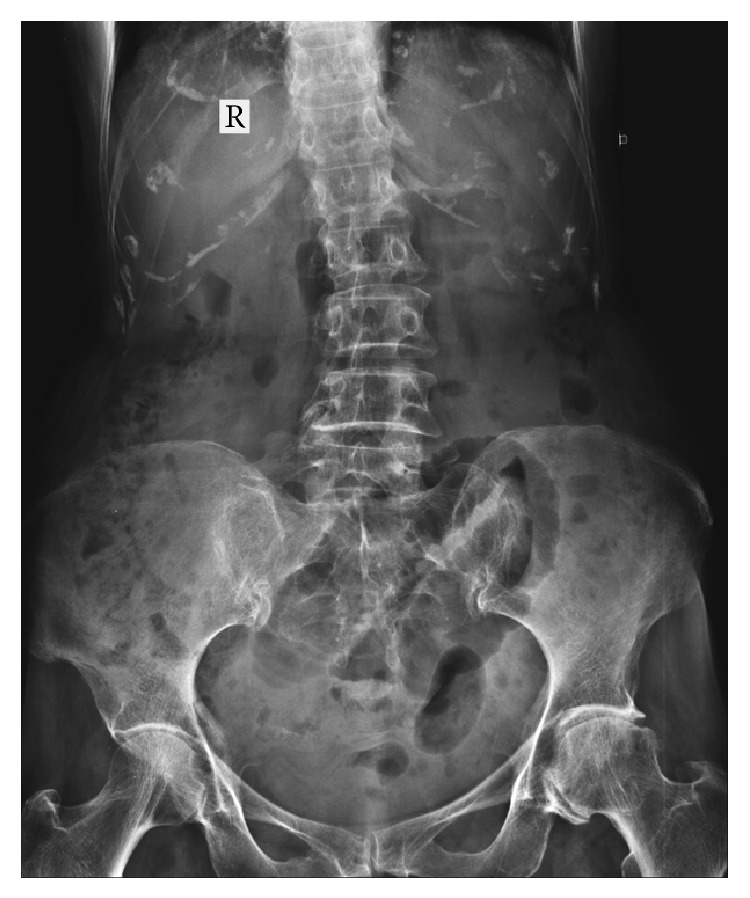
X-ray standing abdomen showing dilated small bowel loops in lower abdomen and pelvis.
